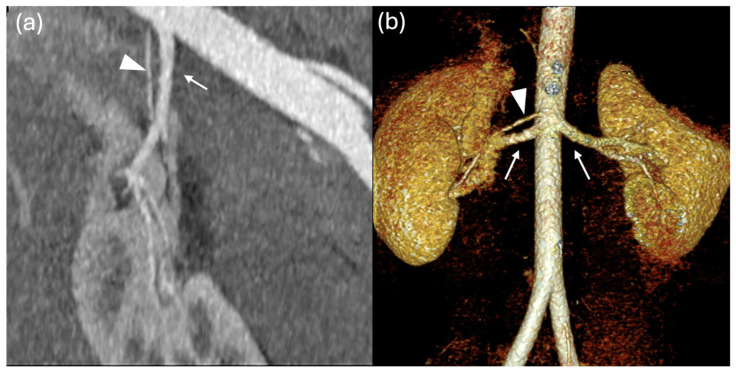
Coronal Maximum Image Projection reconstruction (MIPr) (a) and volume rendering (b) CT images show the main renal arteries (arrows) and an accessory artery (arrowhead) directed to the inferior pole of the right kidney.
